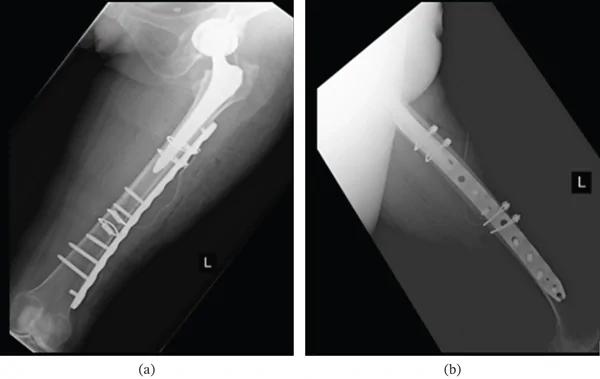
Radiographs obtained following internal fixation of a periprosthetic femoral fracture. (a) Oblique radiograph showing open reduction and internal fixation using a long locking plate and cerclage cables spanning the femoral shaft. (b) Lateral radiograph confirming appropriate alignment and implant positioning without evidence of hardware failure.
